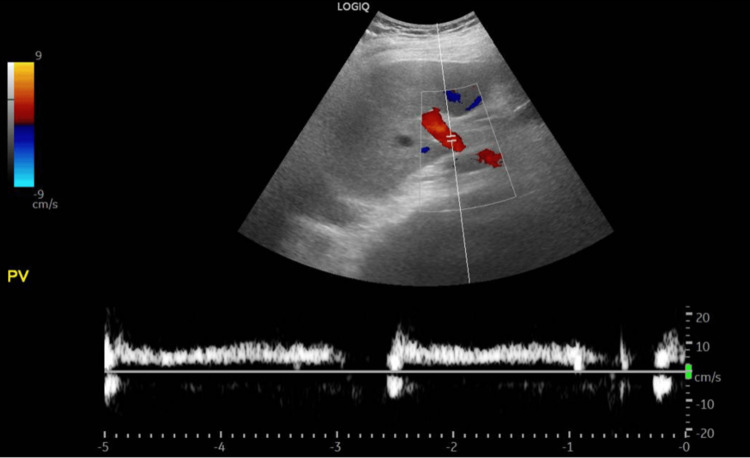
Liver ultrasound with Doppler. Grayscale and Doppler ultrasound images of the liver demonstrating normal hepatic parenchyma and normal hepatic vascular flow.
